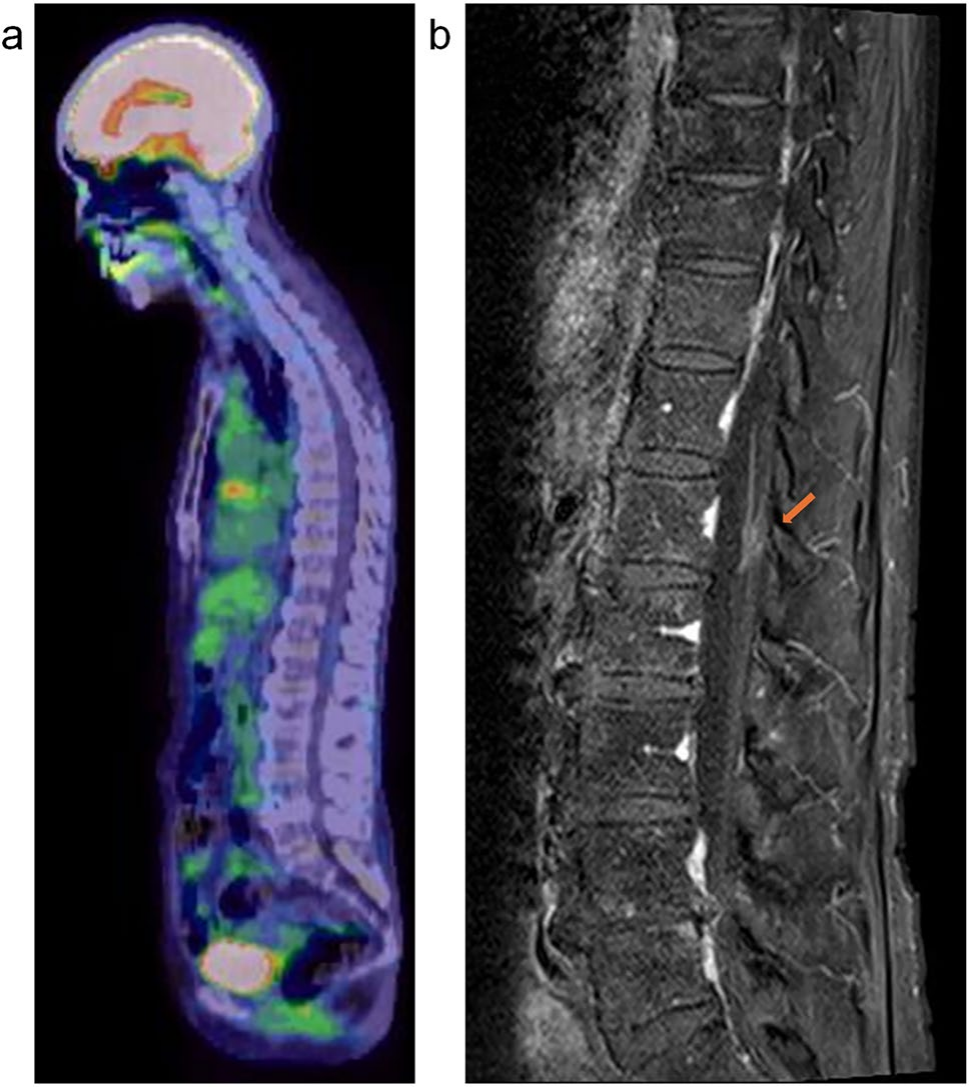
a FDG-PET/CT taken 4 months after radiotherapy showed that FDG accumulation in the ISCM had almost disappeared. b Post-gadolinium T1-weighted MRI images showed a marked shrinkage of ISCM (indicated by arrow in (b); sagittal MRI). *FDG-PET/CT* 18F-fluorodeoxyglucose positron emission tomography/computed tomography; *MRI* magnetic resonance image
